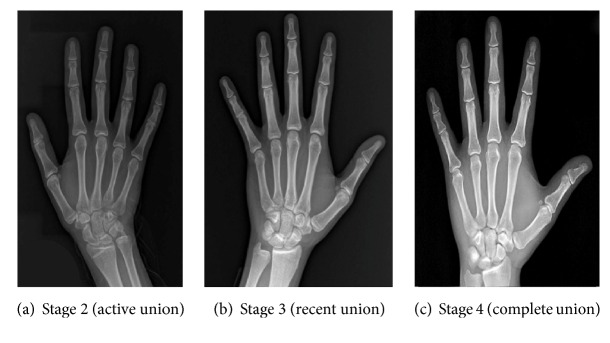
Radiographic images of hand joints showing stage 2 labeled as (a) and stage 3 labeled as (b) and stage 4 labeled as (c).
